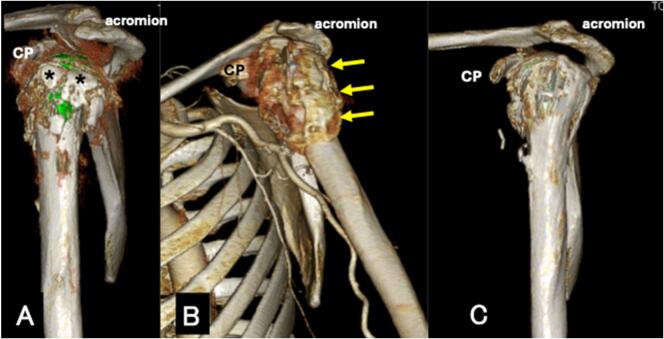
CT scan of the left shoulder immediately after surgery (A), one month (B) and one year (C) after surgery. CP: coracoid process A: Anatomical reduction of the greater tuberosity(asterisk) is seen with cancellous bone graft. B: The callus (arrow) is hardening, indicating that the bone union process is progressing favourably. C: The greater tuberosity fragment is completely healed.
